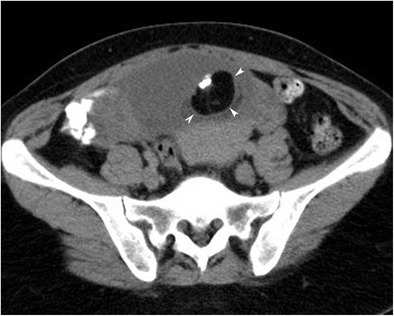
Axial CT image of a 37-year-old female with a mature cystic teratoma shows a rounded Rokitansky nodule (arrowheads) made up of fat and a tooth-like structure in a high-density cystic mass
